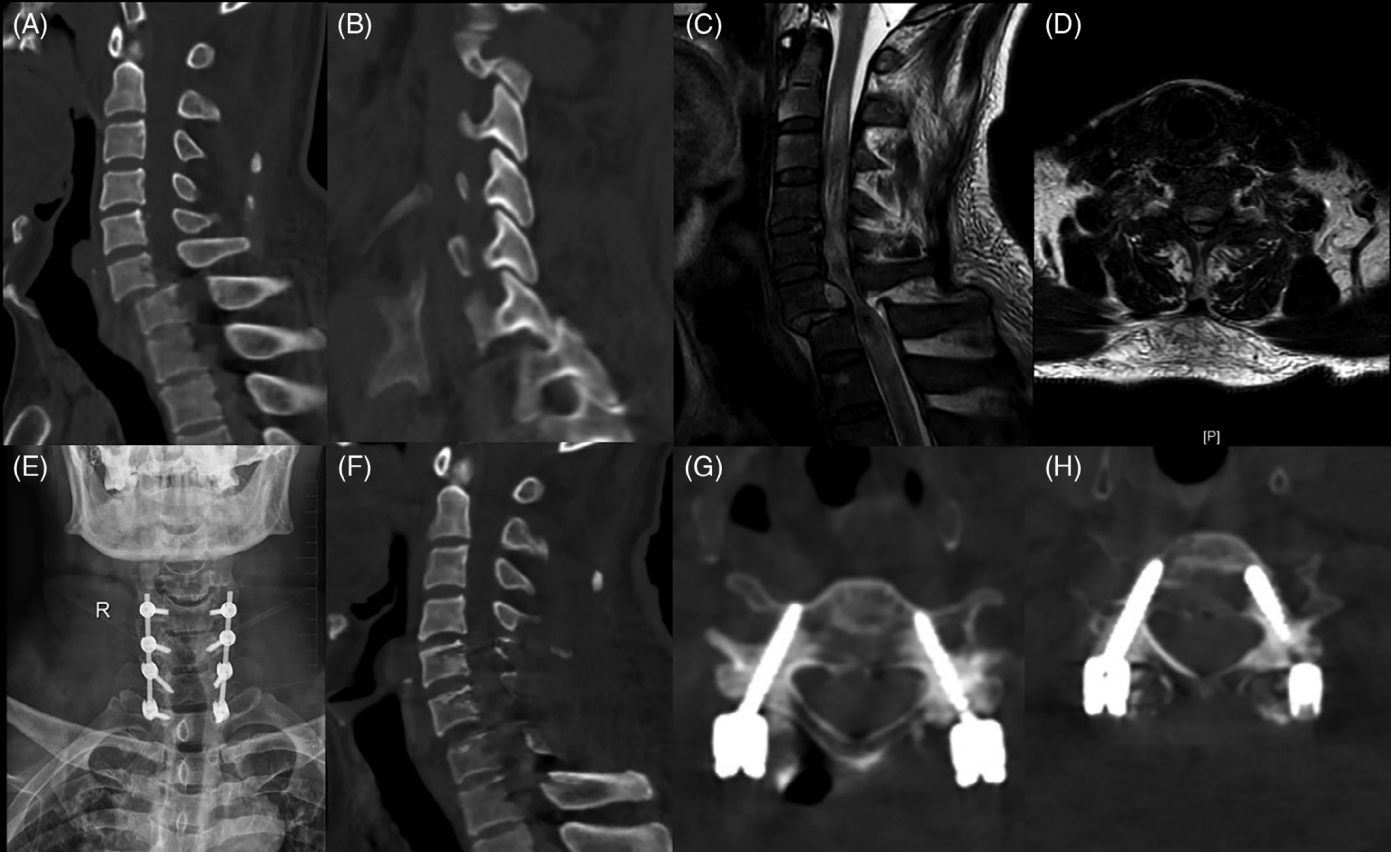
A 55‐year‐old male patient with C_6,7_ fracture‐dislocation undergoing cervical pedicle screw (CPS) fixation treatment with O‐arm navigation. (A‐D) Preoperative CT and MRI showed C_6,7_ fracture‐dislocation with bilateral locked facets and the spinal cord was obviously compressed. (E–H) Postoperative X‐ray and CT showed complete reduction and decompression for the cervical spine and the CPSs were all placed accurately in cervical pedicles.
